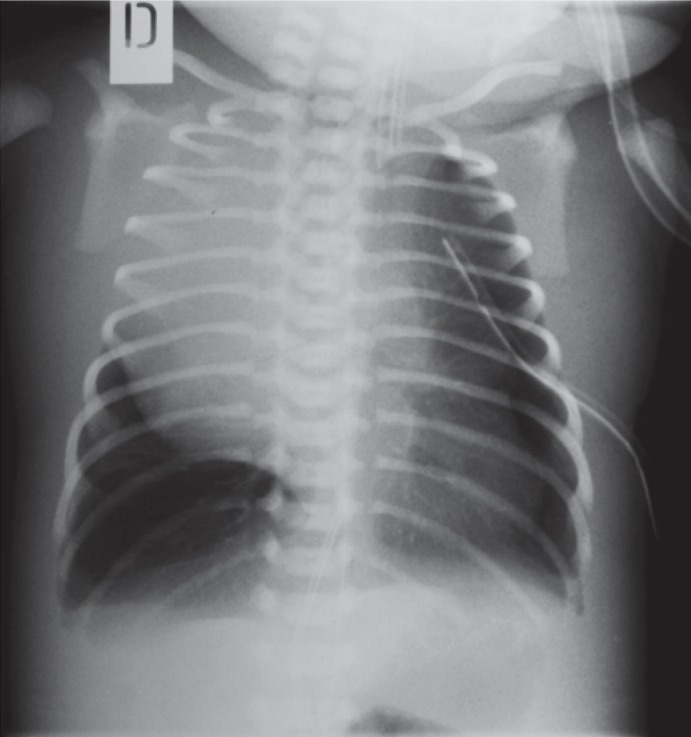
Chest x-ray after birth demonstrates large round tumour in the right hemithorax. (6 × 7 cm) with deviation of mediastinal structures to the left. Soft tissues of the thoracic wall are oedematous.
